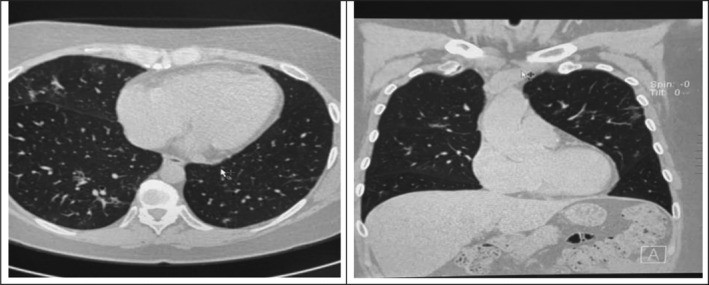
CT after a month of the disease
